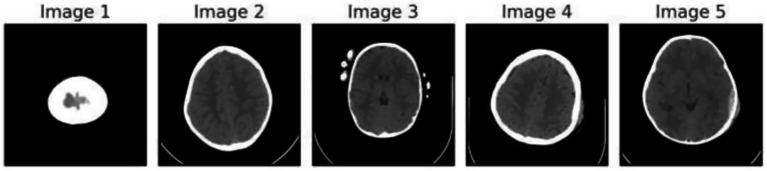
CT scan cross-sections of brain showing stroke variants.
